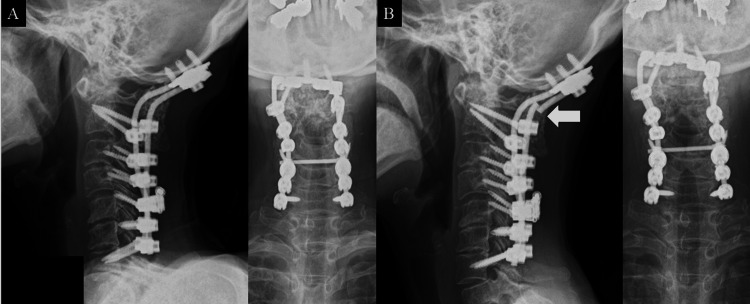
Case 2 (A) X-ray image after primary surgery (Oc-C7 fusion). (B) X-ray image obtained six months after the primary surgery shows unilateral rod breakage (arrow).
